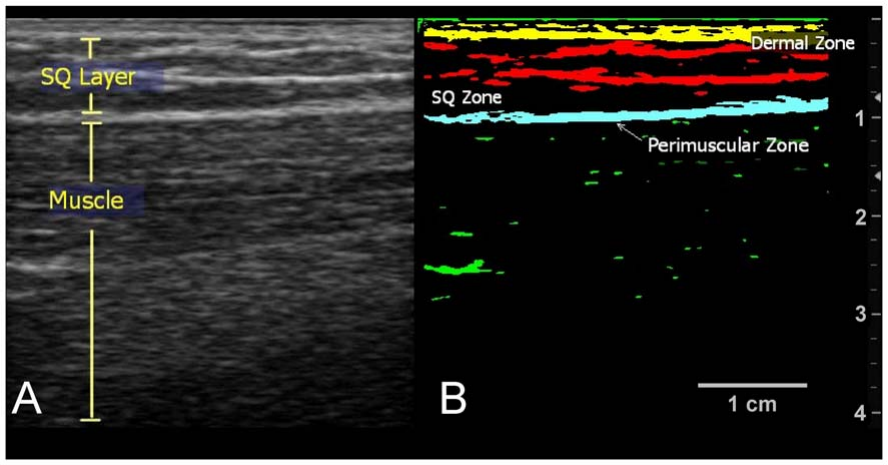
Delineation of Tissue Layers and Zones. (3A.) Ultrasound image. This longitudinal image of the thigh shows the subcutaneous and muscle layers. Echogenic bands (white bands) are seen in the subcutaneous layer. (3B.) Thresholded image. Thresholding the image delineates the echogenic bands in the dermal, SQ, and perimuscular zones.
